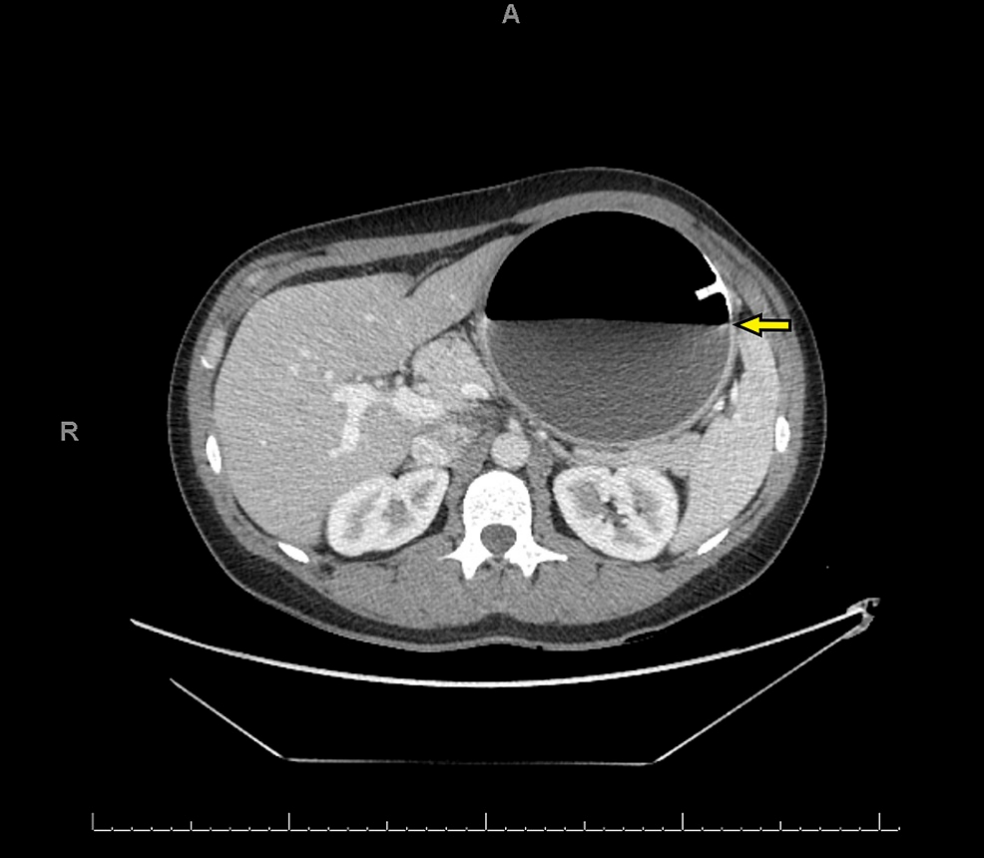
Transverse CT image showing hyperinflated fluid and gas filled IGB. The air fluid level is indicated with an arrow. IGB, intragastric balloon
